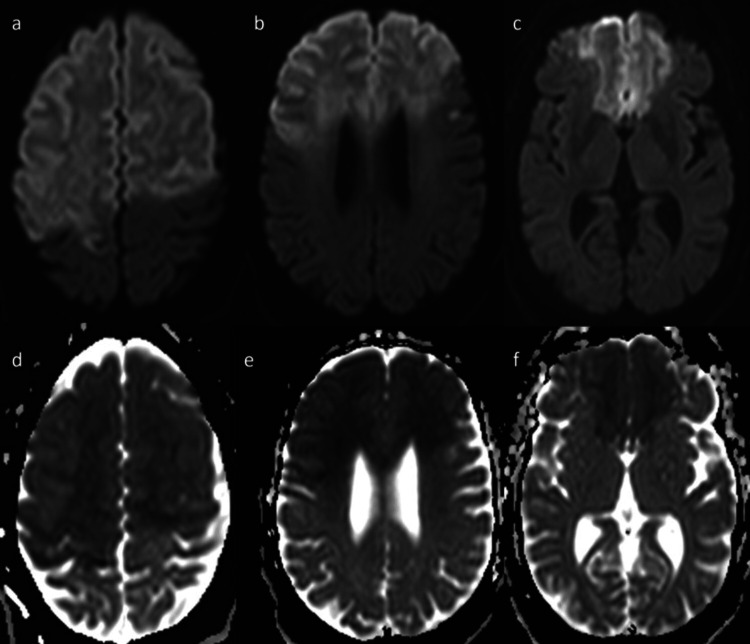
Brain MRI shows confluent early subacute infarcts involving bilateral frontal lobes and right parietal lobe with relative sparing of the inferolateral aspect, and involvement of caudate nuclei. (a) Brain MRI axial diffusion-weighted imaging (DWI) shows confluent hyperintense signal with mild swelling involving bilateral frontal lobes and anterior portion of right parietal lobe. (b) Brain MRI axial DWI shows confluent hyperintense signal with mild swelling involving bilateral frontal lobes, with relative sparing of the inferolateral aspect. (c) Brain MRI axial DWI shows confluent hyperintense signal with mild swelling involving bilateral frontal lobes with relative sparing of the inferolateral aspect, and involvement of caudate nuclei. (d, e, f) MRI brain ADC shows corresponding low signal intensity.
